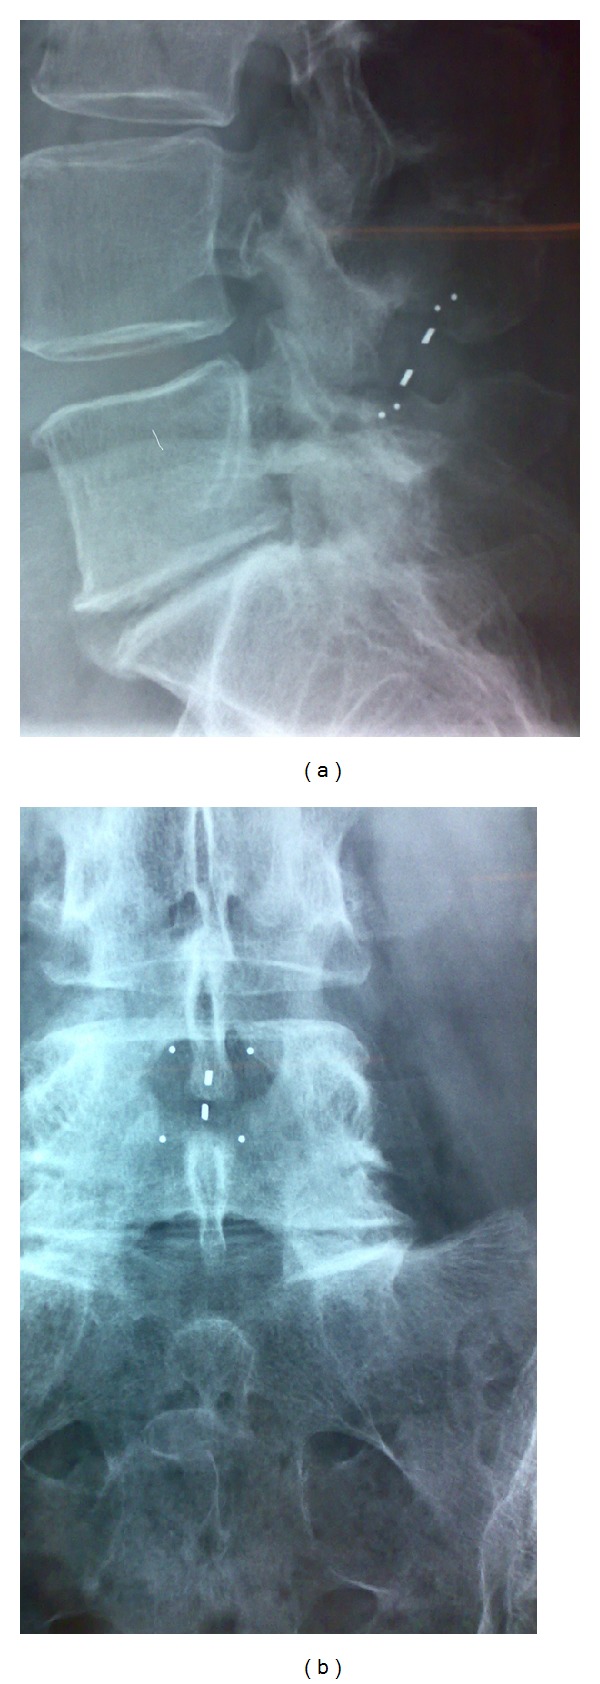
12 months after procedure laterolateral (a) and anteroposterior (b) radiographs are useful to demonstrate the correct position of this L4-L5 implant.
